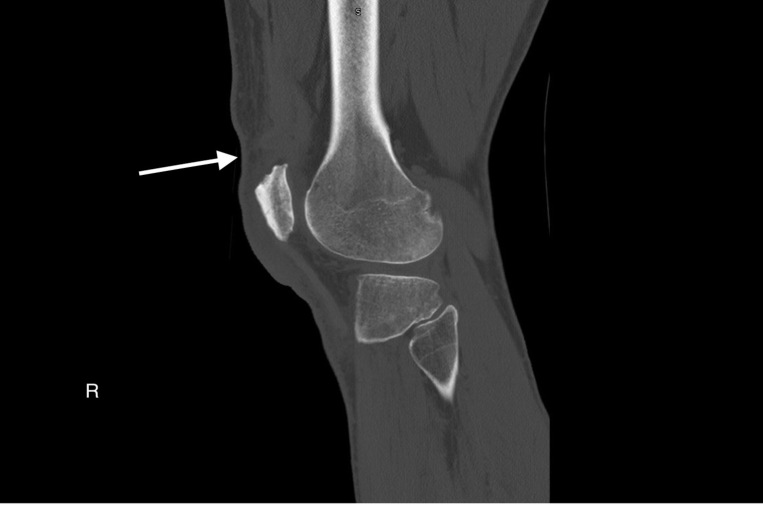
Computed tomography of right knee demonstrating near-complete tear of quadriceps tendon with retraction of the central portion of the tendon (arrow).
